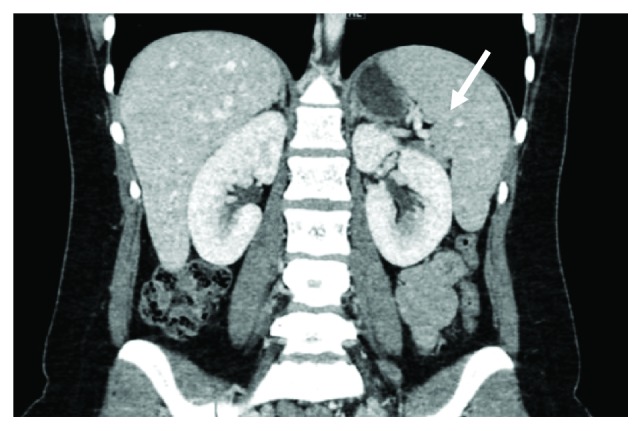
Baseline CT scan shows the normal size and appearance of the spleen before using granulocyte colony-stimulating factor (G-CSF).
